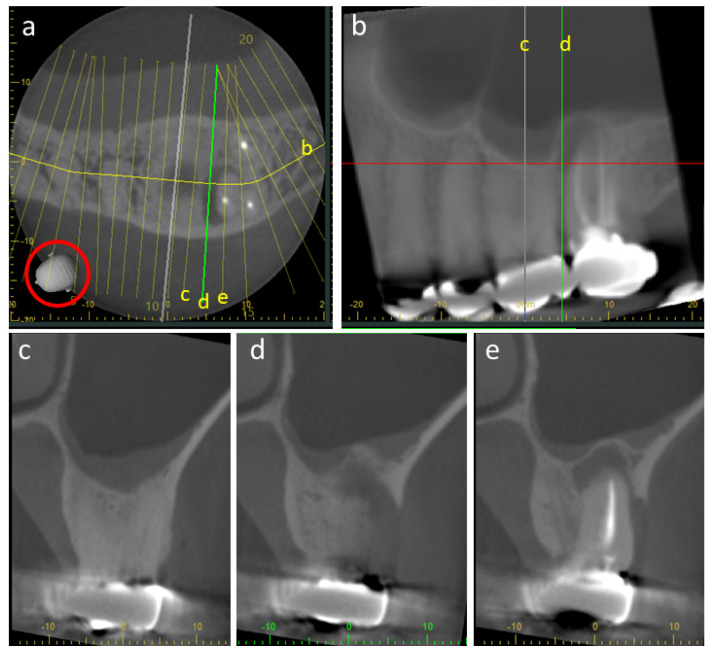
Depicted is the same 4 cm × 4 cm FOV CBCT dataset as in Figure 6 but displayed in a curved/panoramic multiplanar reconstruction: (a) is axial; (b) is panoramic (the white line in (a)) and (c–e) are transaxial; and (d) is the green line in (a), which are immediately before (c) and behind (e). The ‘head’ displays the orientation they are all constructed from.
